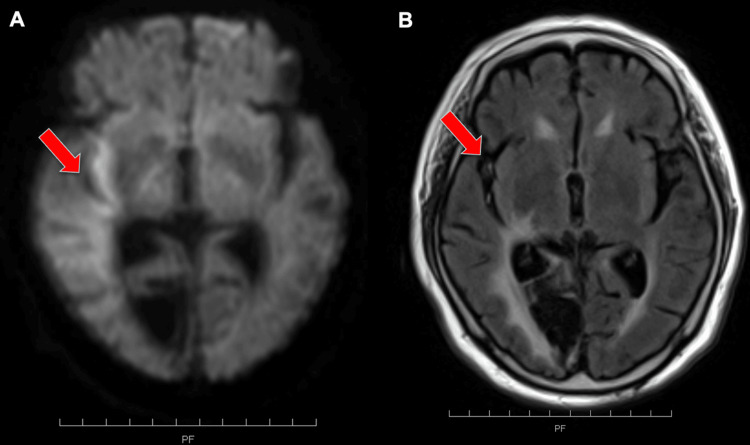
MRI brain of cerebral infarction Diffusion-weighted magnetic resonance imaging (DWI) revealed an acute infarct in the right MCA inferior trunk region (A: arrow), although the image was unclear because of motion artifacts. FLAIR images showed an intra-arterial sign in the middle cerebral artery (MCA) inferior trunk region (B: arrow). FLAIR: fluid attenuated inversion recovery
